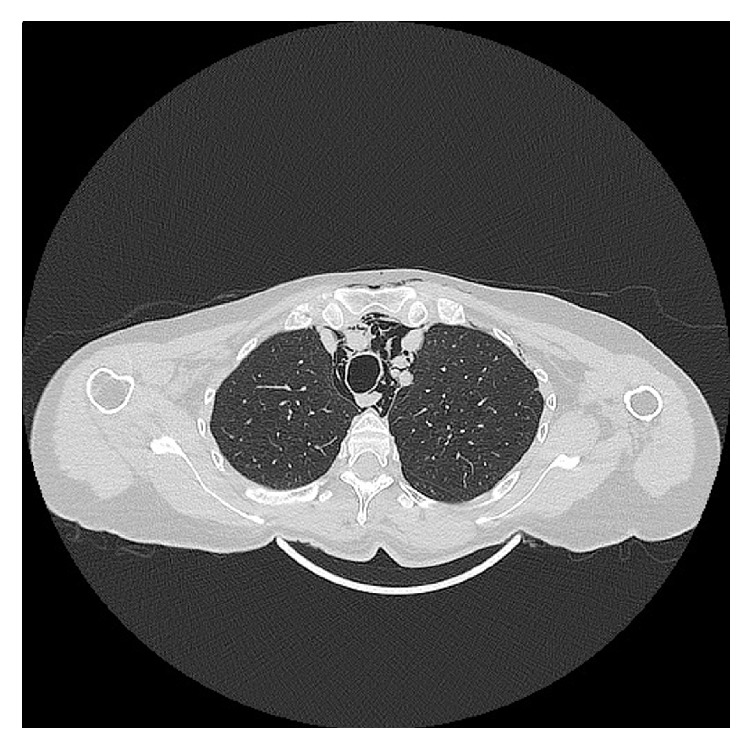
Computed tomographic axial view of the emphysema descending to the mediastinum, where it is evident mainly in the anterior region of perivascular adiposity and surrounding the trachea and oesophagus posteriorly.
